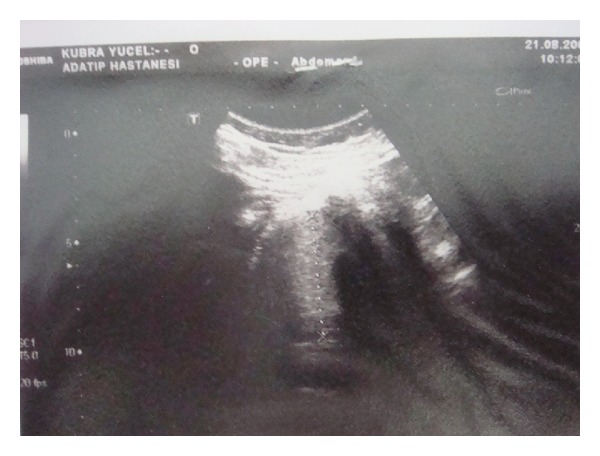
(Ultrasonographic (USG) examination): the lesion well-defined, solid, hyperechoic mass of pancreatic head-body, measuring 5 × 5 cm. No infiltration into the intra-abdominal organs is seen.
